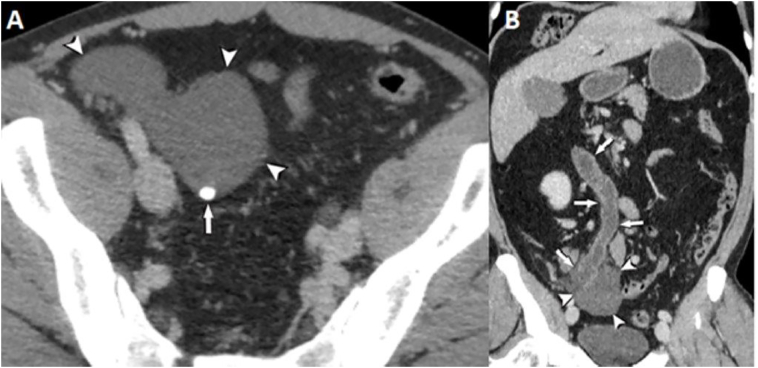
A + B: Axial (A) and coronal (B) enhanced CT images show localized fluid in the right lower abdominal quadrant (arrowheads in A and B) with a solitary calcification (arrow in A). The proximal slightly fluid-distended jejunal intestine loop (arrows in B) has an atypical straight orientation towards the right lower abdomen with swirling mesentery vessels suspicious for an internal hernia.
